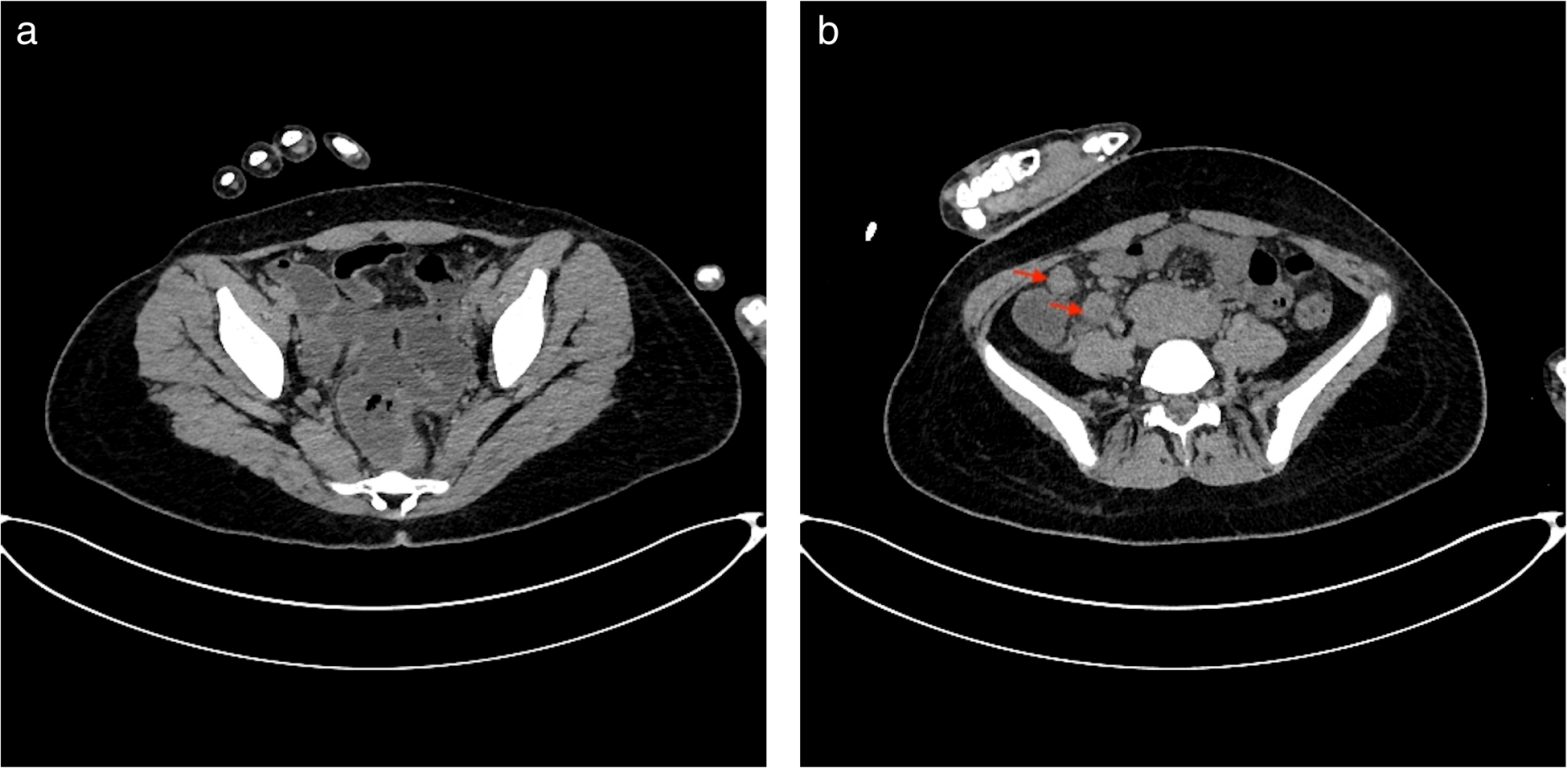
Abdominal computed tomography showing (a) slight thickening of the rectosigmoid colon, (b) multiple enlarged lymph nodes in the right lower quadrant
